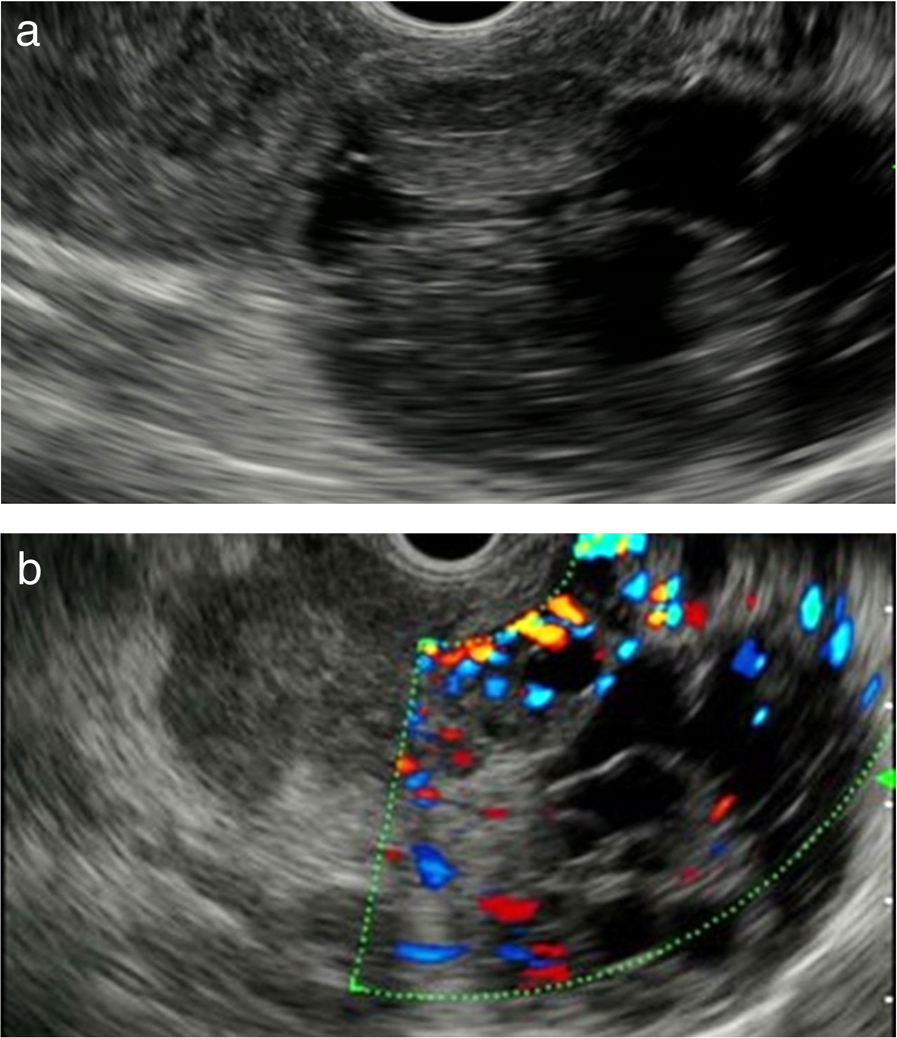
Endoscopic ultrasonography (EUS) findings. a EUS revealed a well-demarcated cystic mass with solid components in the pancreas tail. b Color Doppler EUS revealed the tumor was hypervascular, particularly on the surface
